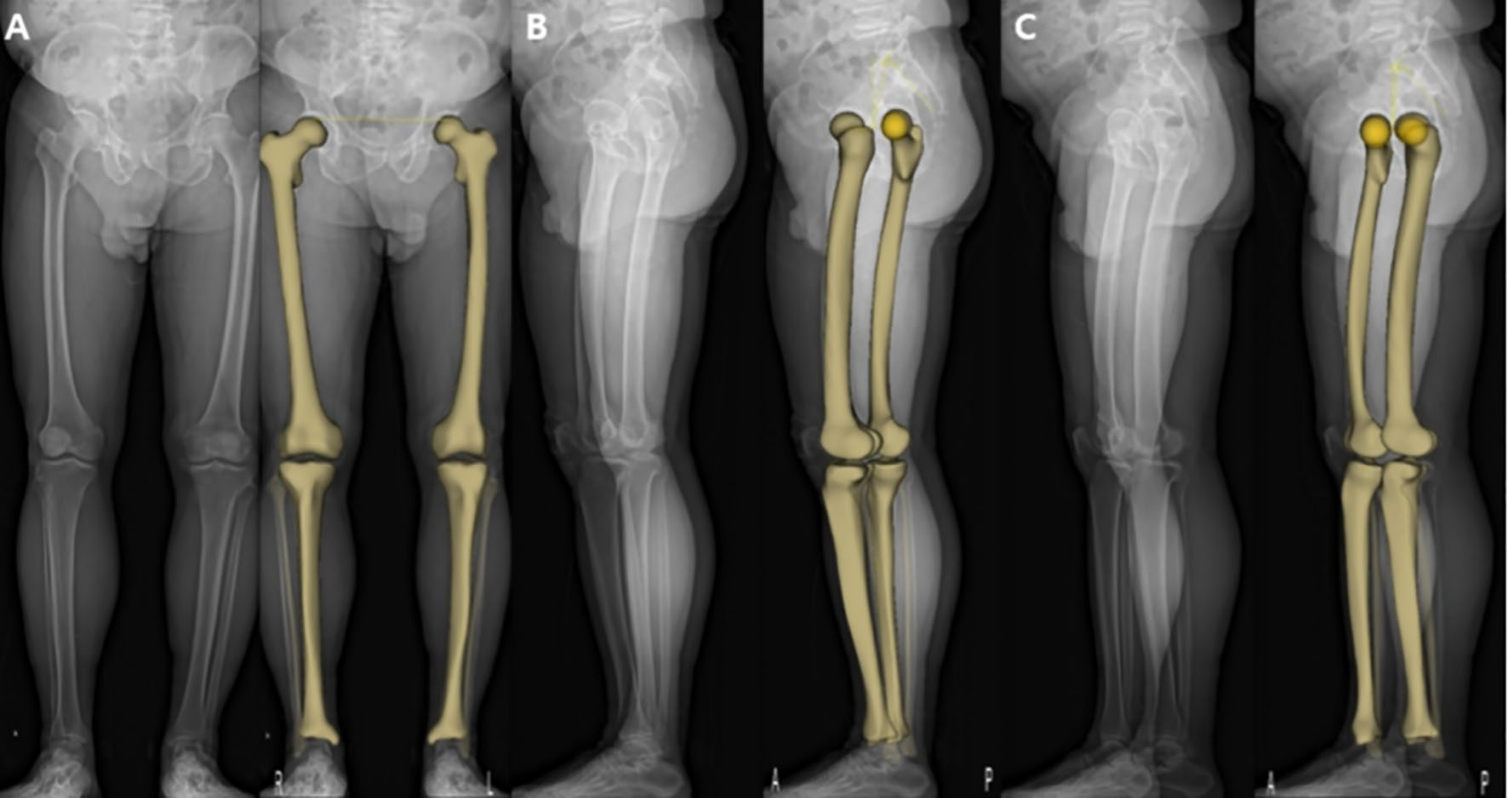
Three-dimensional (3D) model based on the EOS imaging system. Anteroposterior (A) and lateral radiographs (B and C) are acquired for 3D modeling. The 3D model is adapted to the osseous contours on radiographs.
